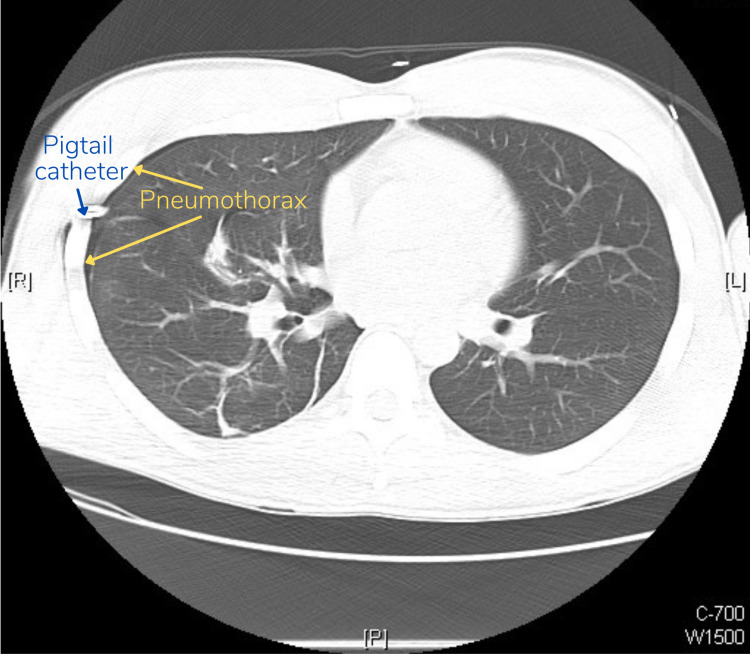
Chest CT performed three hours after initial presentation demonstrating small, residual pneumothorax (5%) following pigtail catheter placement
